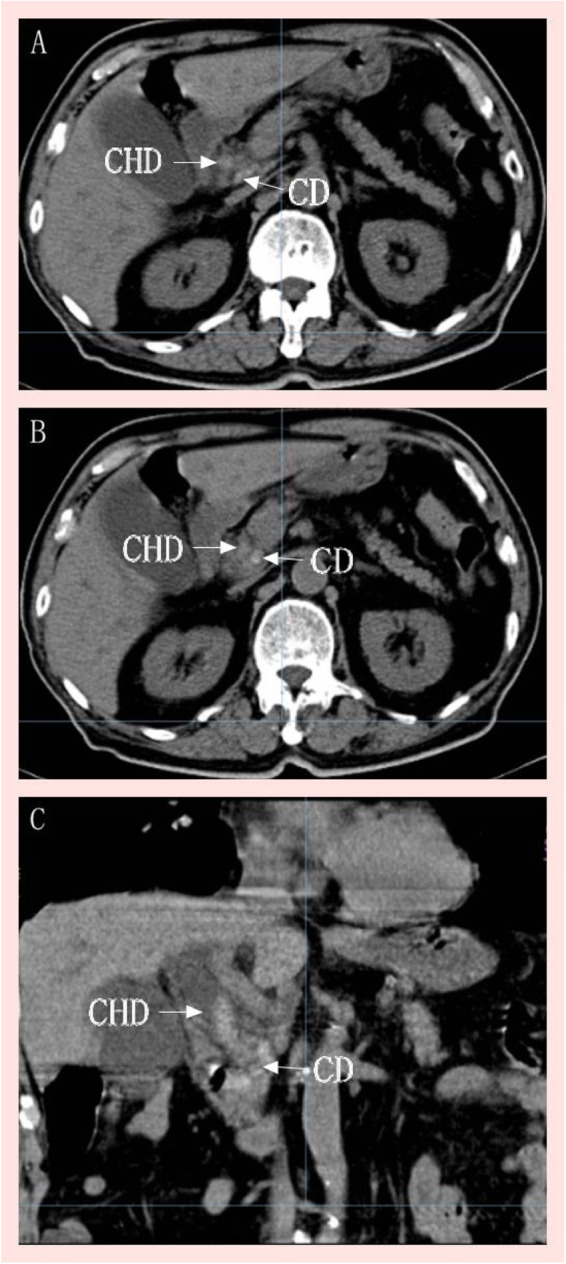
Preoperative upper abdominal CT: multiple stones in the bile duct and CD, with marked dilation of the extrahepatic bile duct; panels (A) and (B) demonstrate the CD coursing dorsally from the common hepatic duct to the left side, running parallel to the common hepatic duct. Panel (C) reveals the low confluence of the CD into the bile duct. CD, cystic duct; CHD, common hepatic duct.
